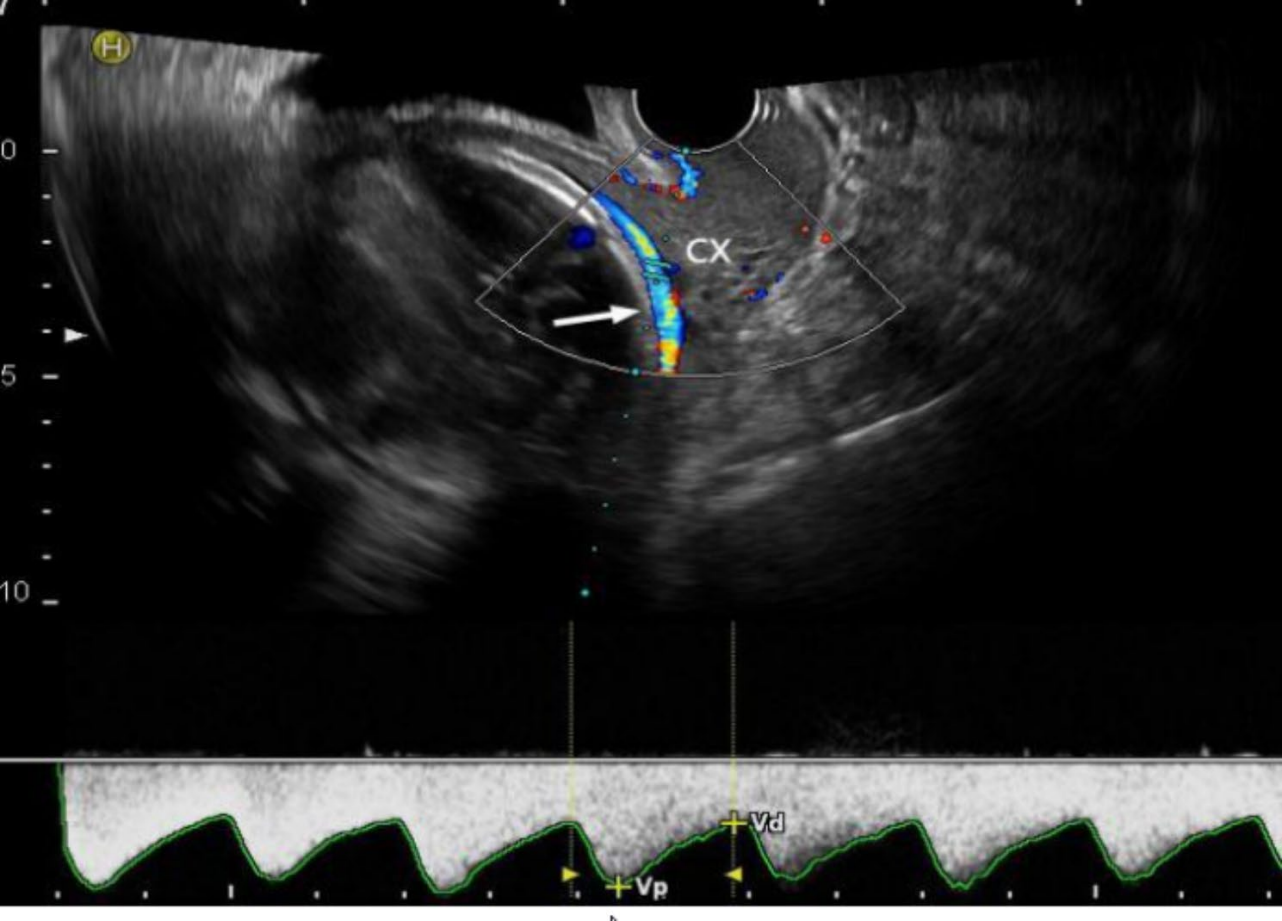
The umbilical cord insertion showed by sonographic scanning in case 1. White arrow indicates the umbilical insertion on the fetal membranes and overlay of the internal cervical os with arterial waveform observed above the internal cervical os at 32 weeks of gestation
